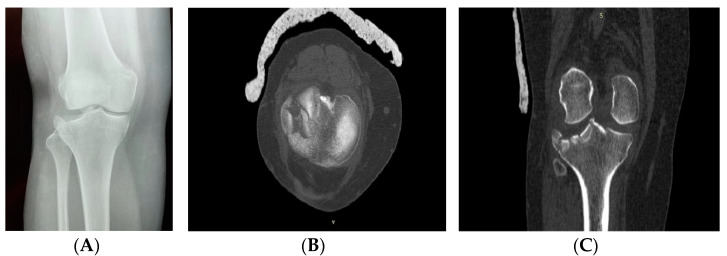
Pre-operative imagistic investigations of the right knee. (A) Anteroposterior plain radiographs of the right knee showing lateral tibial plateau fracture. (B) Initial CT scan coronal plan of the right knee showing the comminution of the lateral tibial plateau. (C) Initial CT scan axal slice of the right knee showing the impression of the fracture in the lateral tibial plateau.
